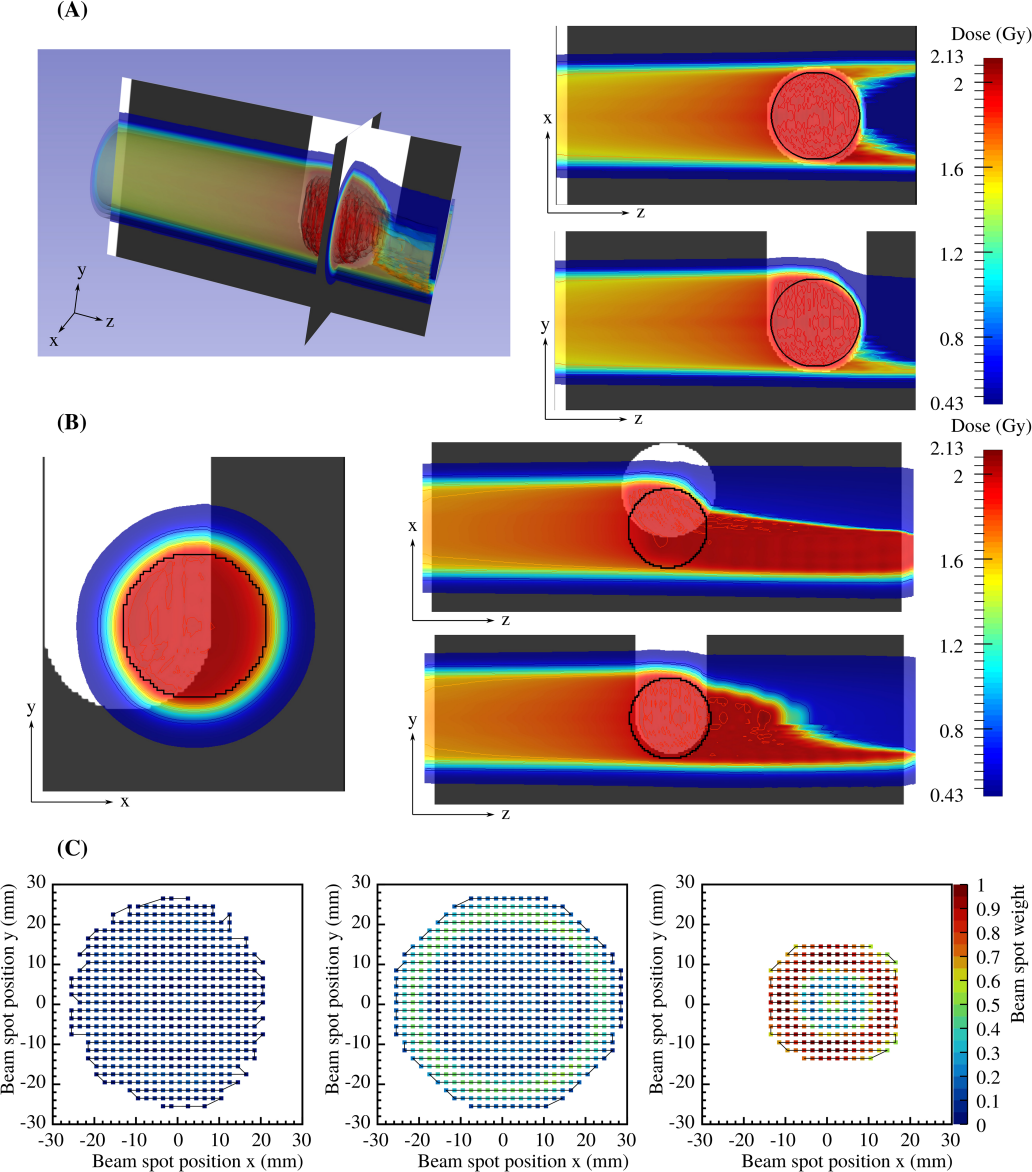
Delivered dose superimposed to the synthetic CT as 2D and 3D views when the treatment is delivered as planned (A), while in (B) the treatment is delivered at a motor position of 20 mm in *x* direction. In (C), *x*-*y* views of three single iso-energy layers that were extracted from the 3D treatment plan for 131.32, 170.11 and 195.65 MeV/u containing 495, 603 and 219 beam spots, respectively. The black line shows the trajectory of the irradiation, and the particles per beam spot were normalized to the maximum value of the 195.65 MeV/u treatment plan.
